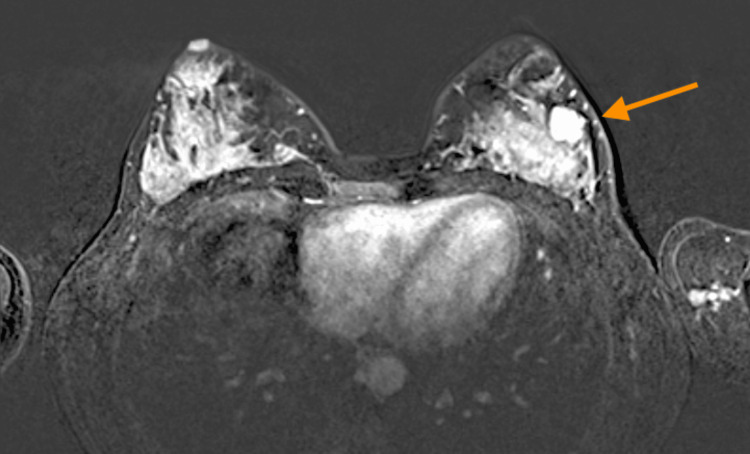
The MRI showed a mass (arrow) with early enhancement after paramagnetic contrast administration. MRI: magnetic resonance imaging
